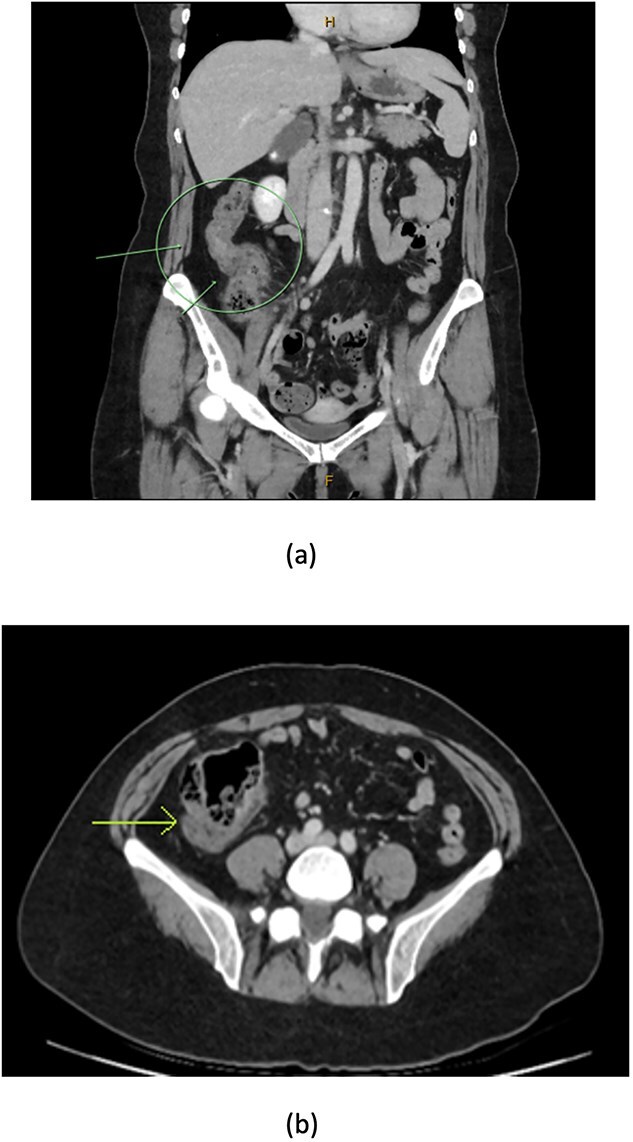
CT coronal (a) and axial (b) views showing thickening of the ascending colon (arrows) with faecalised and distended distal ileum.
